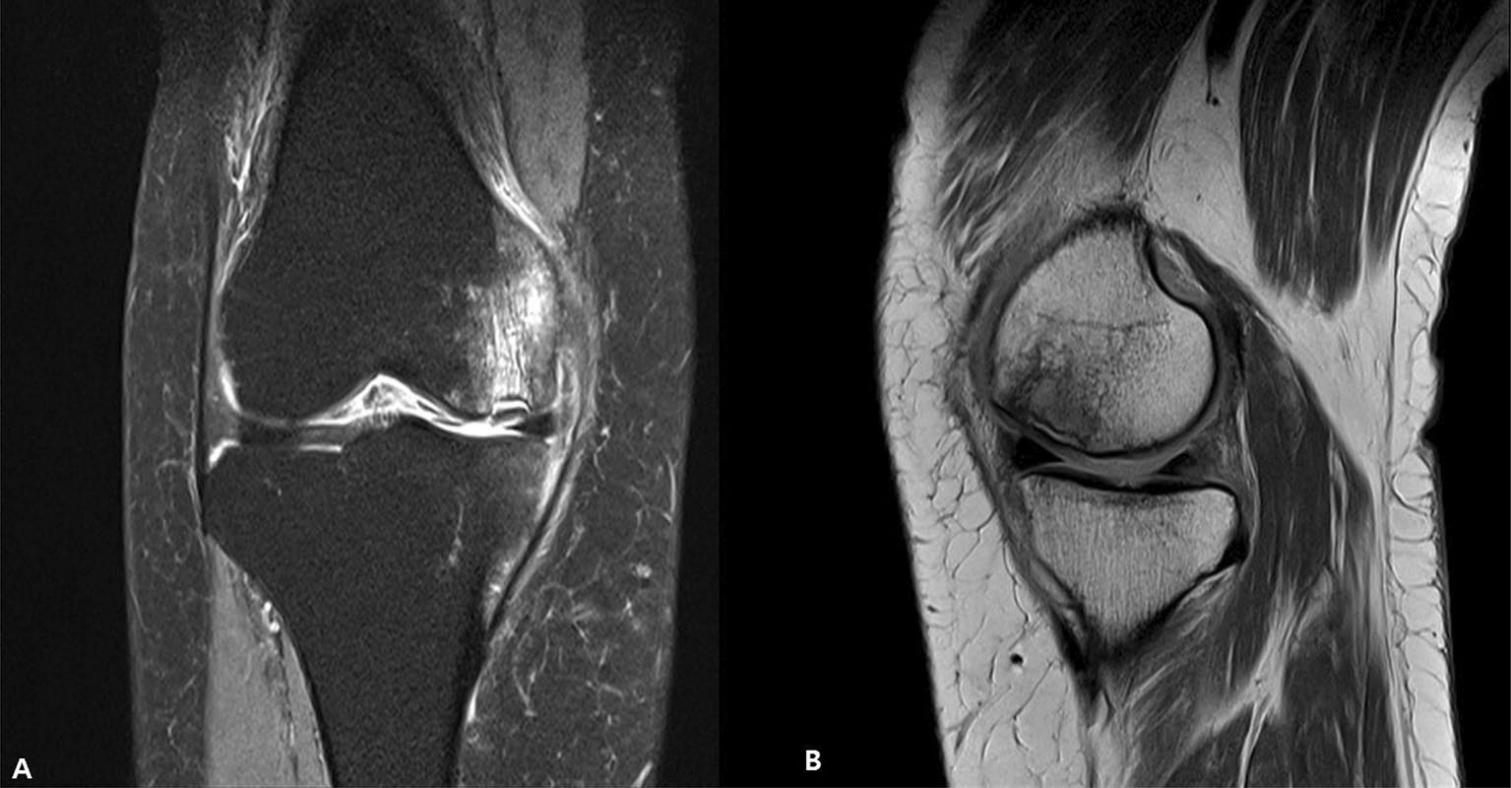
Coronal T2-weighted MRI of SIFK (left). Sagittal T1-weighted MRI (right). MRI, magnetic resonance image. SIFK, subchondral insufficiency fracture of the knee.
